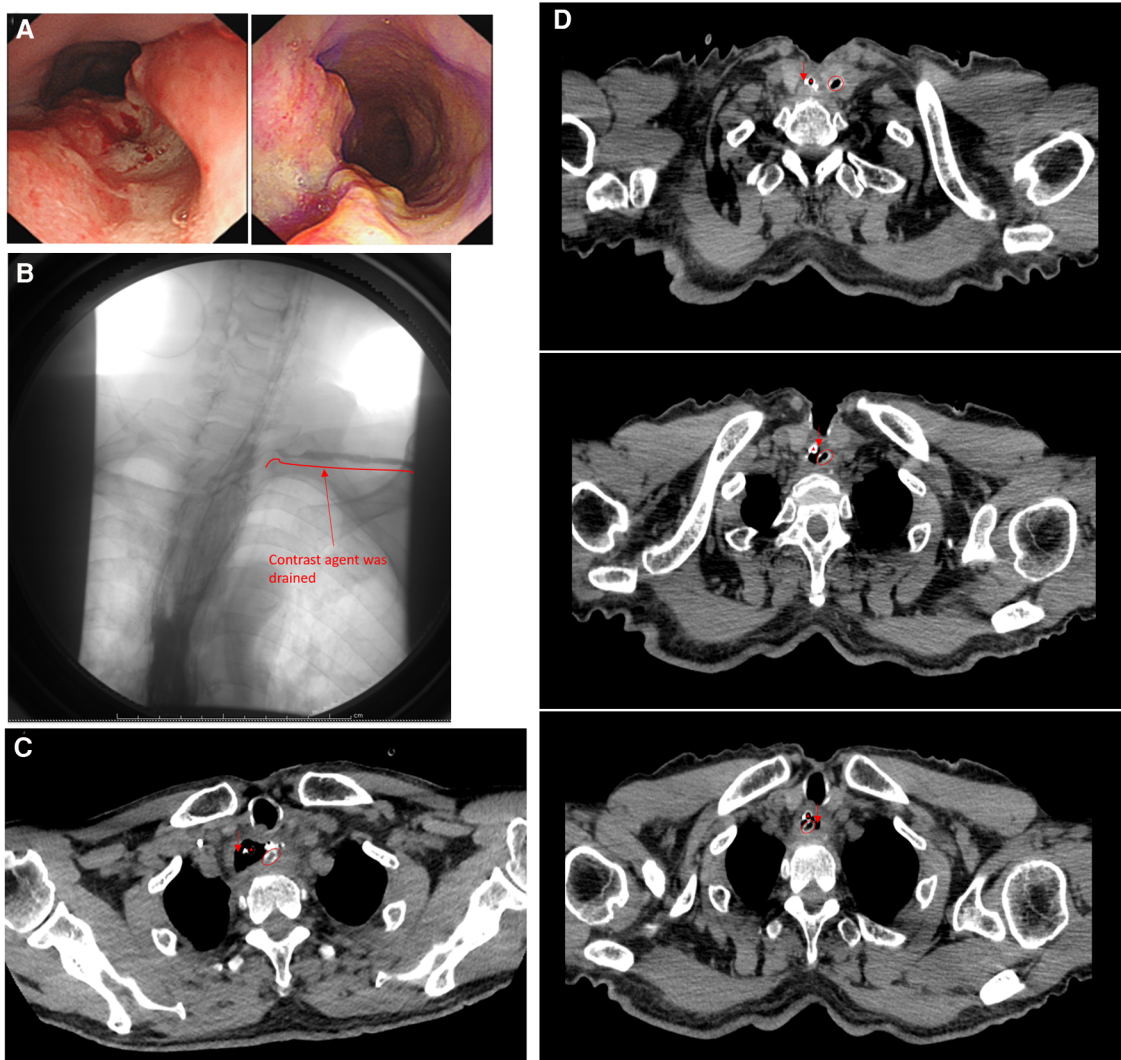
(A) Preoperative gastroscopic pictures of the patient; (B) esophagography on POD 57, contrast agent was drained through the cervical drainage tube; (C) CT on POD 8, the cervical drainage clung to the esophageal wall but not penetrate into; (D) three slices of CT images on POD 12 showed the extend of the cervical drainage tube from the cervical wound to the esophageal cavity. ○, cervical drainage tube; ▴, gastric tube; ↓, esophageal cavity.
